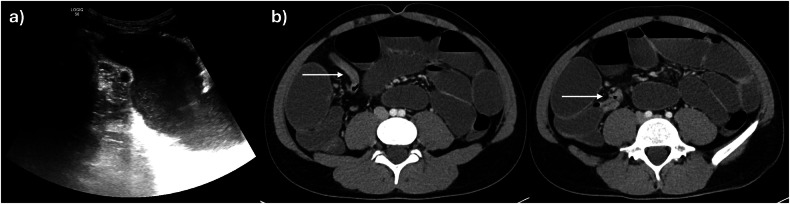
Twenty-six-year-old male patient with obstructive symptoms presented at the emergency department. a IUS shows dilated, fluid-filled small bowel loops. b Axial emergency contrast-enhanced CT images in portal venous phase confirm small bowel obstruction due to stricturing disease at the level of the terminal ileum (arrows)
